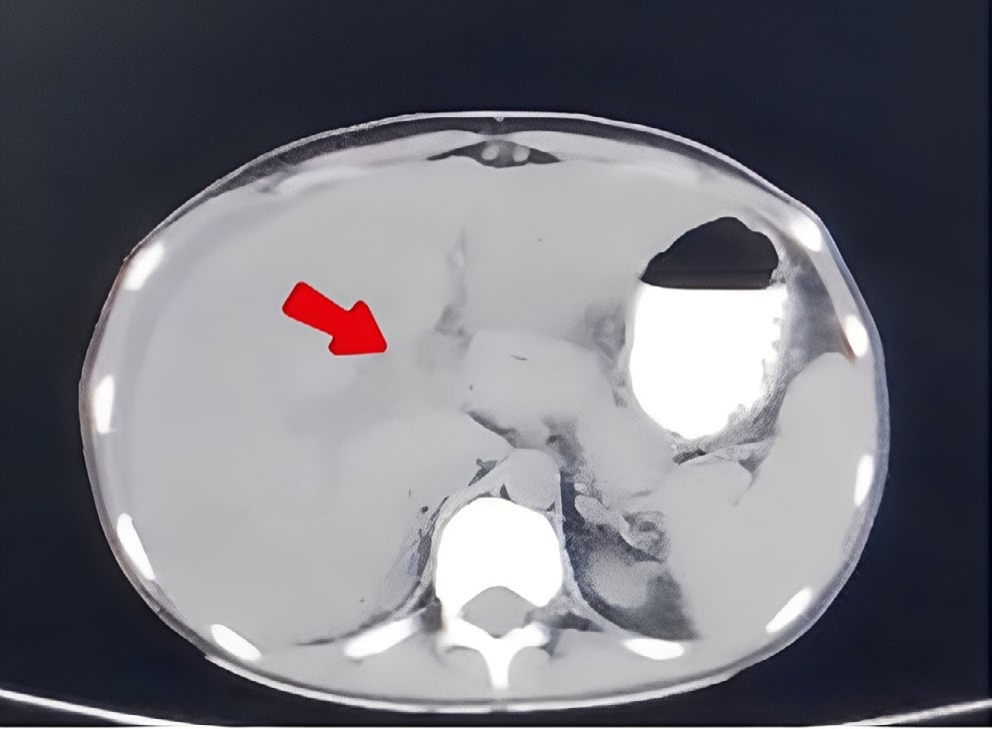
CT abdomen showing early enhancement of the central part of the liver and the caudate lobe around the inferior vena cava (IVC), with evidence of a filling defect in the main portal vein. The right, middle, and left hepatic veins are not visualized. Perihepatic free fluid is seen, suggestive of ascites.
